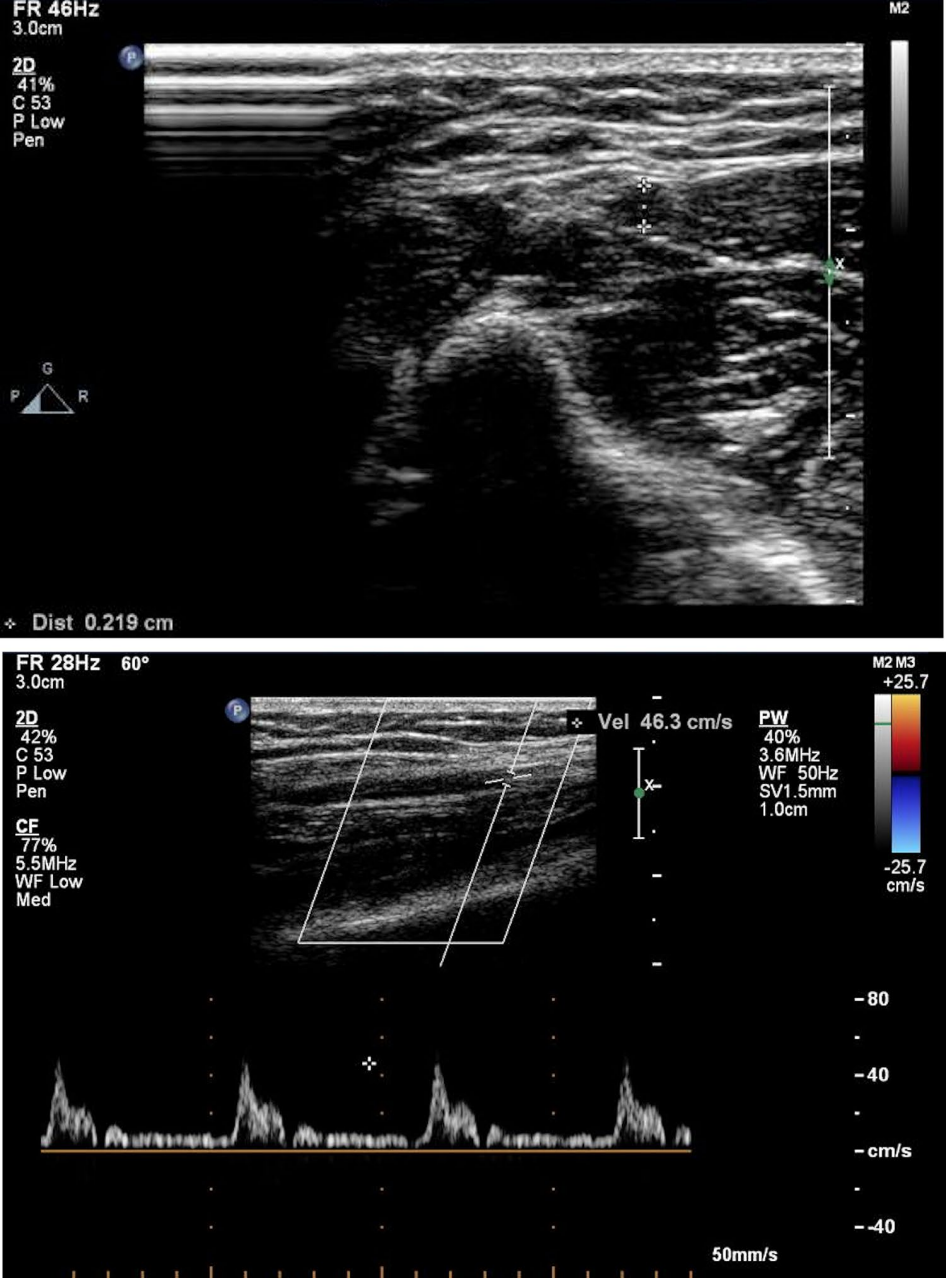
Ergonovine provocation test of radial artery measured by ultrasound. (A) Short axis view indicating the inner diameter of the radial artery. (B) Spectral Doppler indicating the peak systolic velocity of the radial artery.
